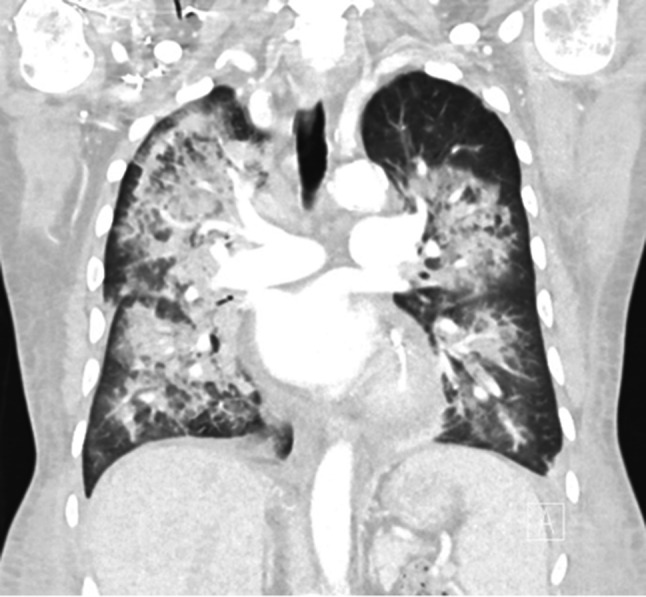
Computed tomography (CT) chest coronial image consistent with vasculitis-associated pulmonary haemorrhage
